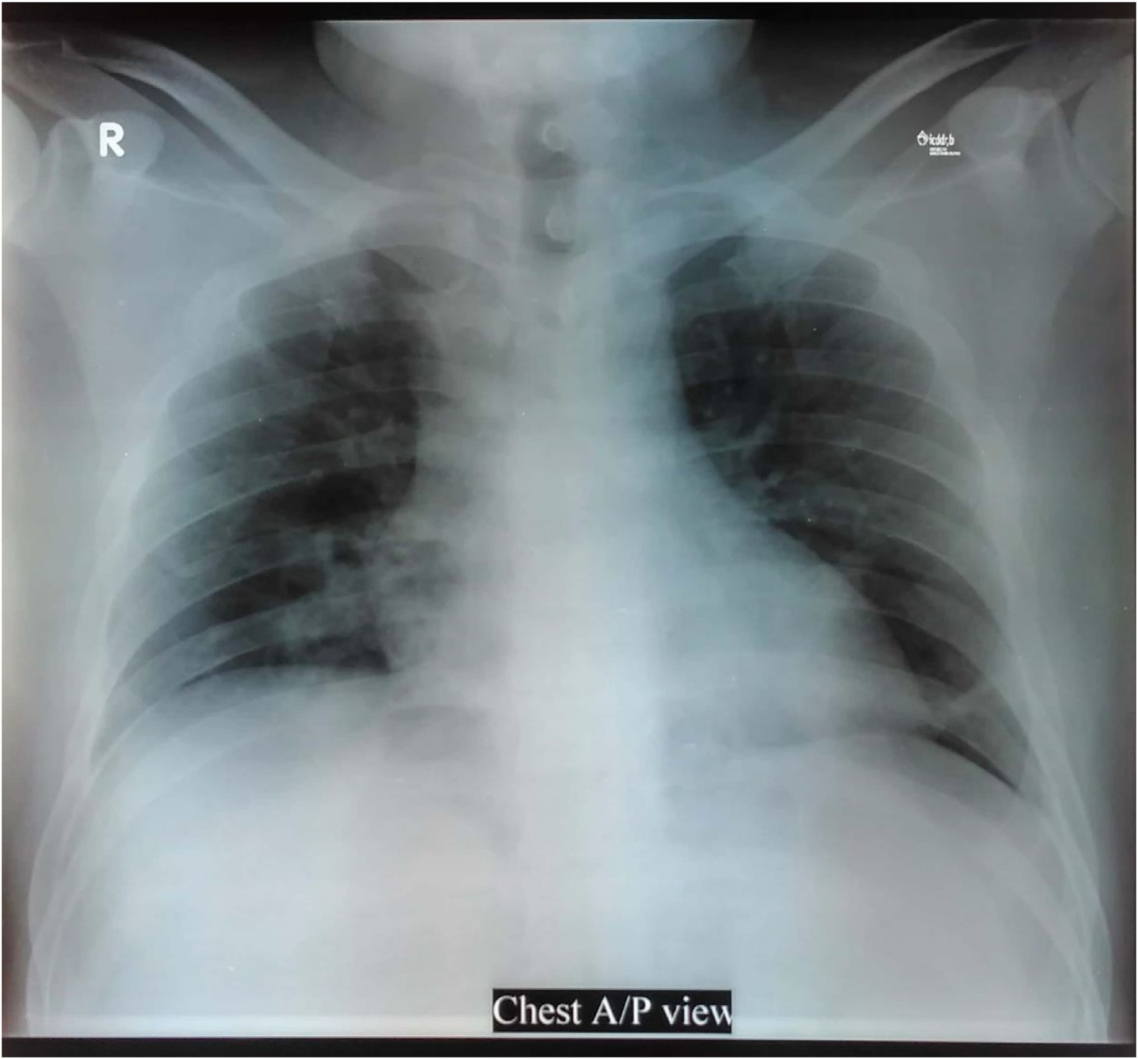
Chest X-ray anteroposterior view showing ill-defined ground-glass opacification (GGO) in the lower left and right lung zone, with an overall score of 2.
